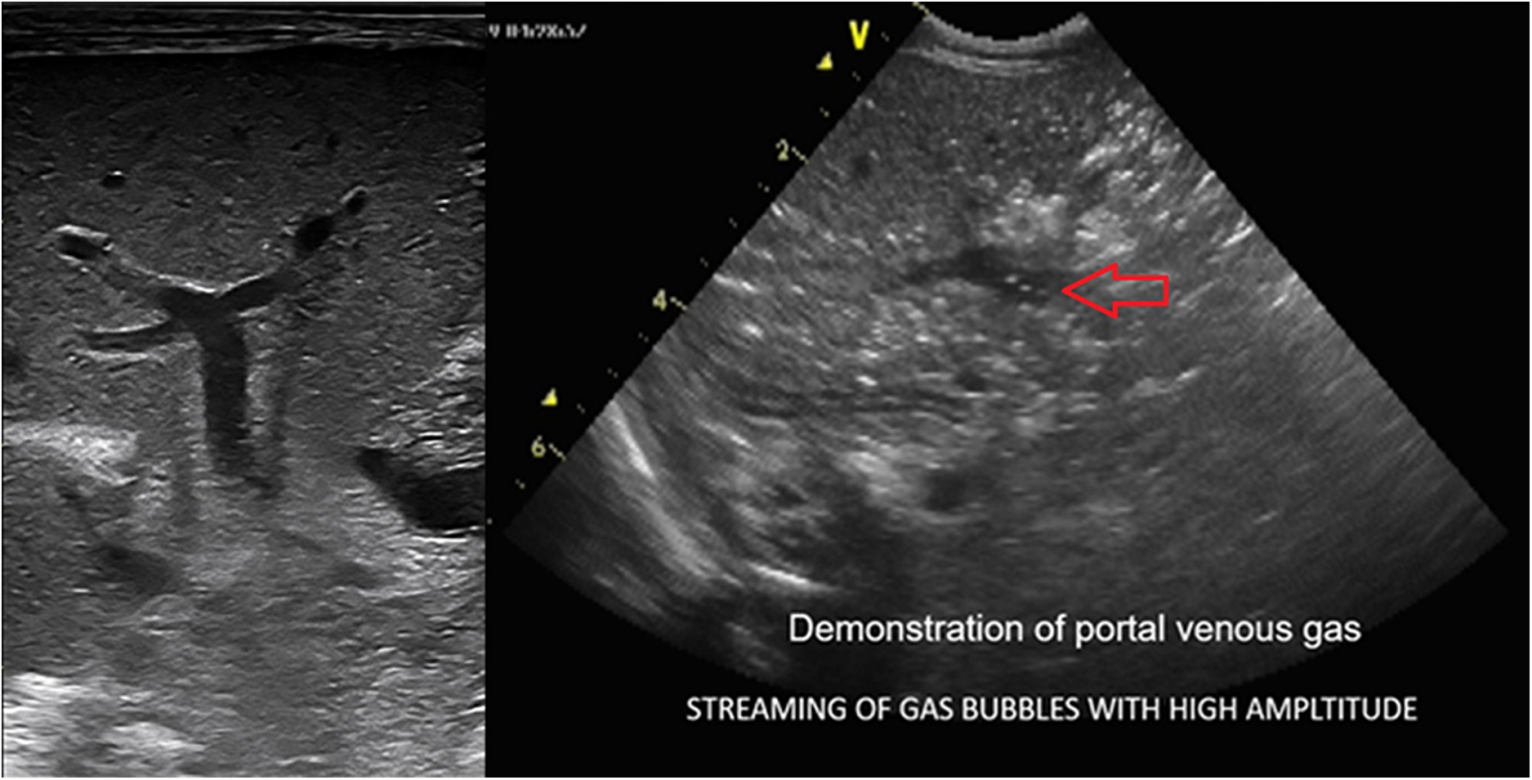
The sonographic appearance demonstrating real-time visualization of portal venous gas release seen as bright echogenic dots (streaming of gas bubbles through the portal vein). The entrapment of these bubbles within the porto-hepatic venous architecture results in abnormal hepatic echotexture seen as interspersed bright echogenic dots as opposed to the normal homogenous hepatic echotexture of a normal Liver.
